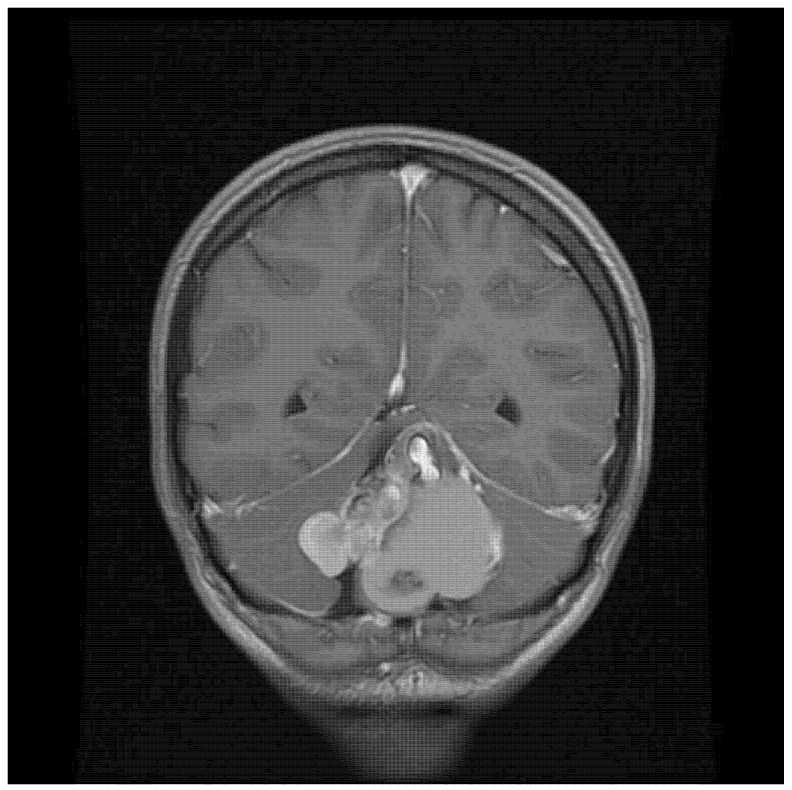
A coronal contrast-enhanced T1-weighted image showing the tumor located between the cerebral hemispheres, with a visible tooth.
